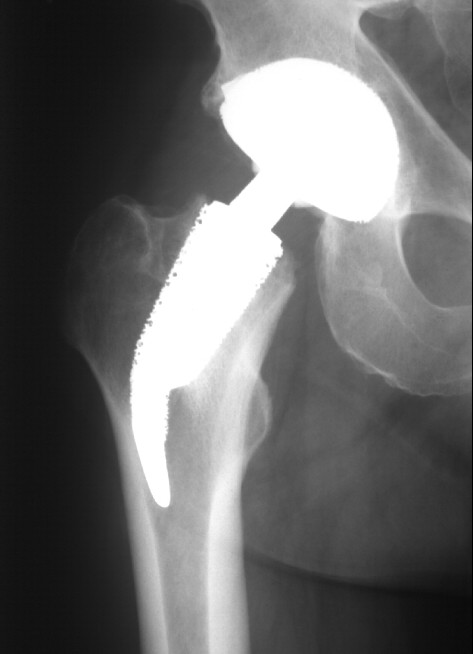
ap radiograph of the Cut prosthesis.
